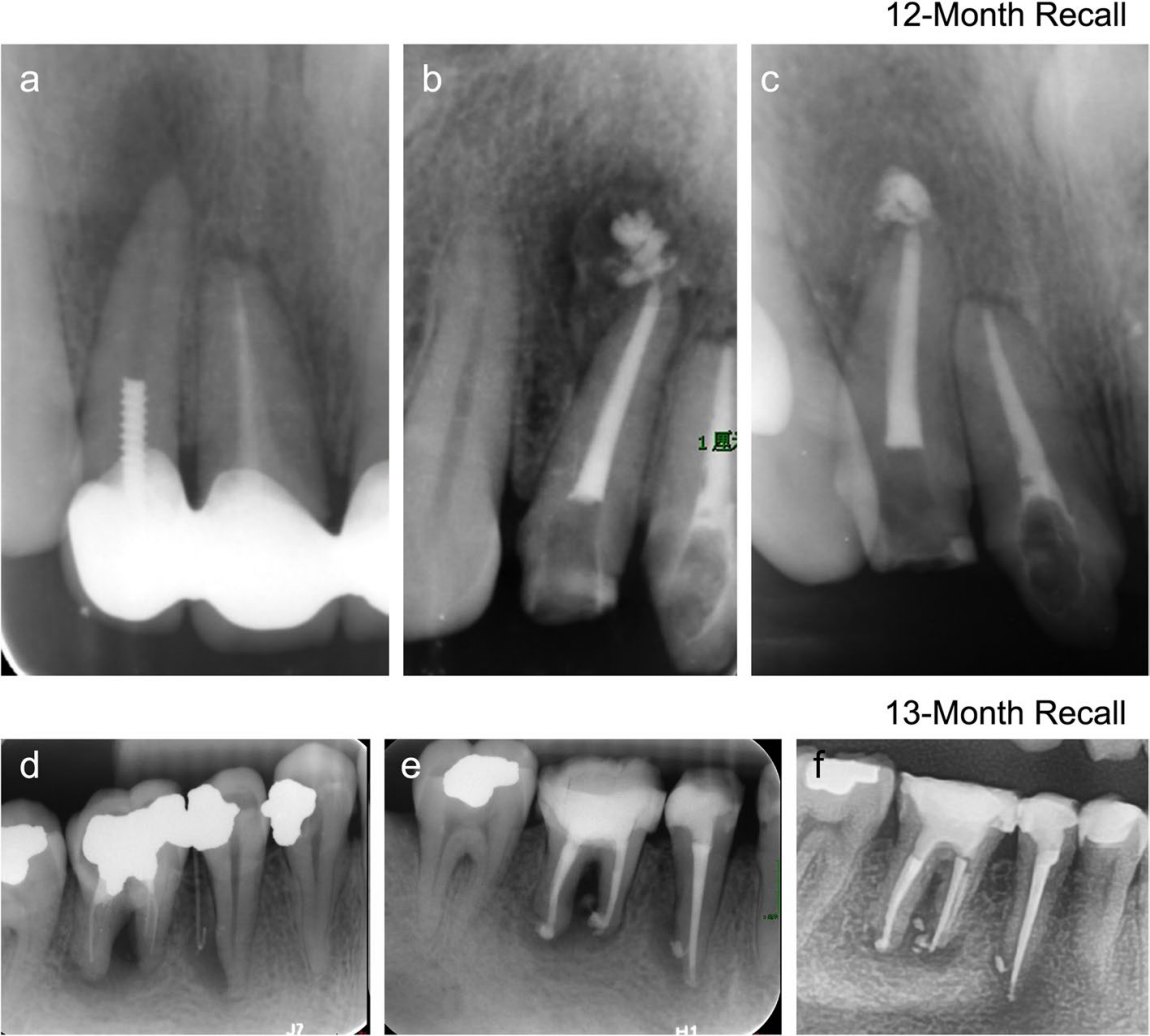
Preoperative (a and d), postoperative (b and e), and recall (c and f) radiographs of outcomes of healing teeth
